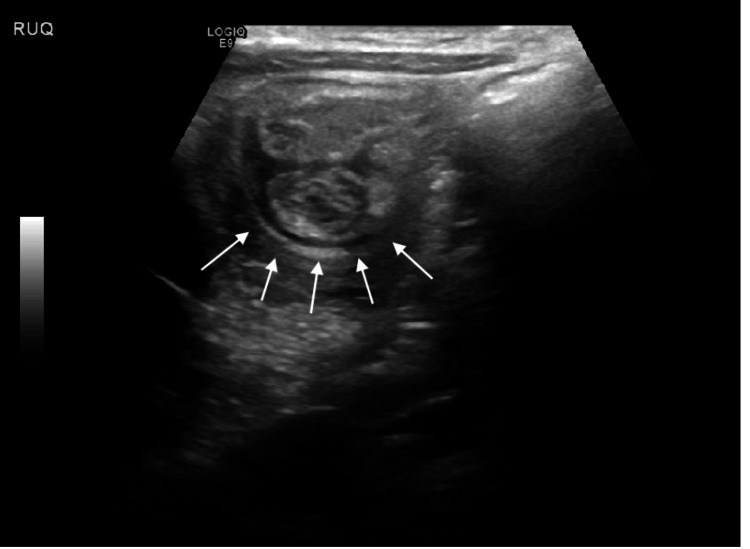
Abdominal ultrasound image at the time of presentation The image shows Ileocolic intussusception at least to the level of the hepatic flexure (white arrows).
